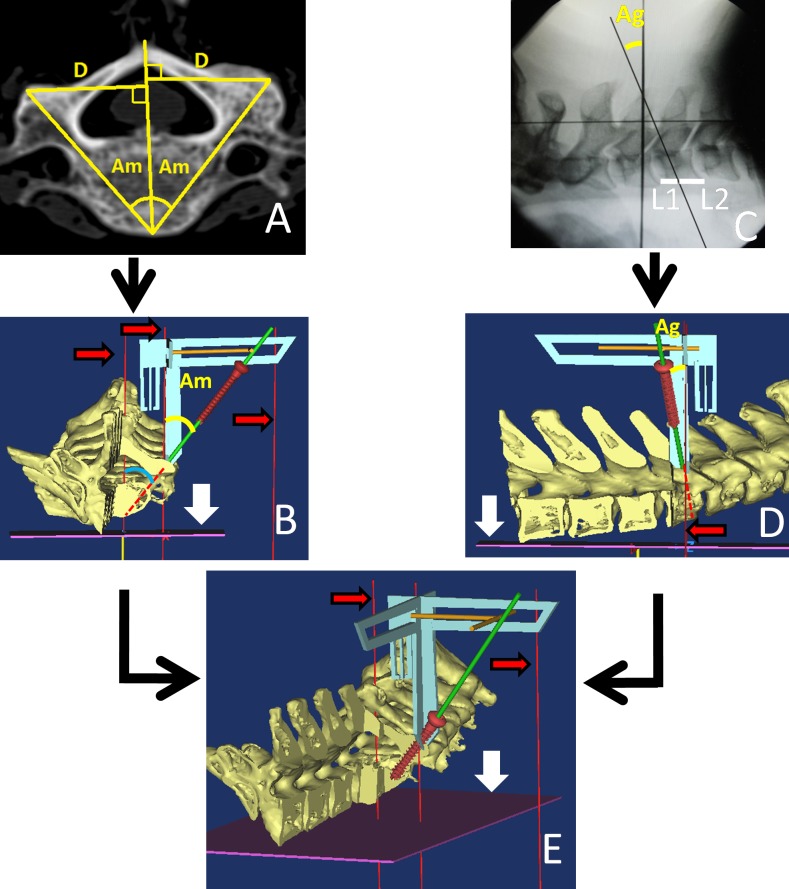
The three-dimensional demonstration of insertion using caliper A and B. (A) Am obtained from the preoperative CT. (B) The realization of Am in the coronal plane. (C) Ag obtained from the intraoperative fluoroscopy. (D) The realization of Ag in the sagittal plane. (E) The ideal insertion of pedicle screw in the appropriate orientation. Red narrow arrow: the gravity line. White broad arrow: the ground plane.
